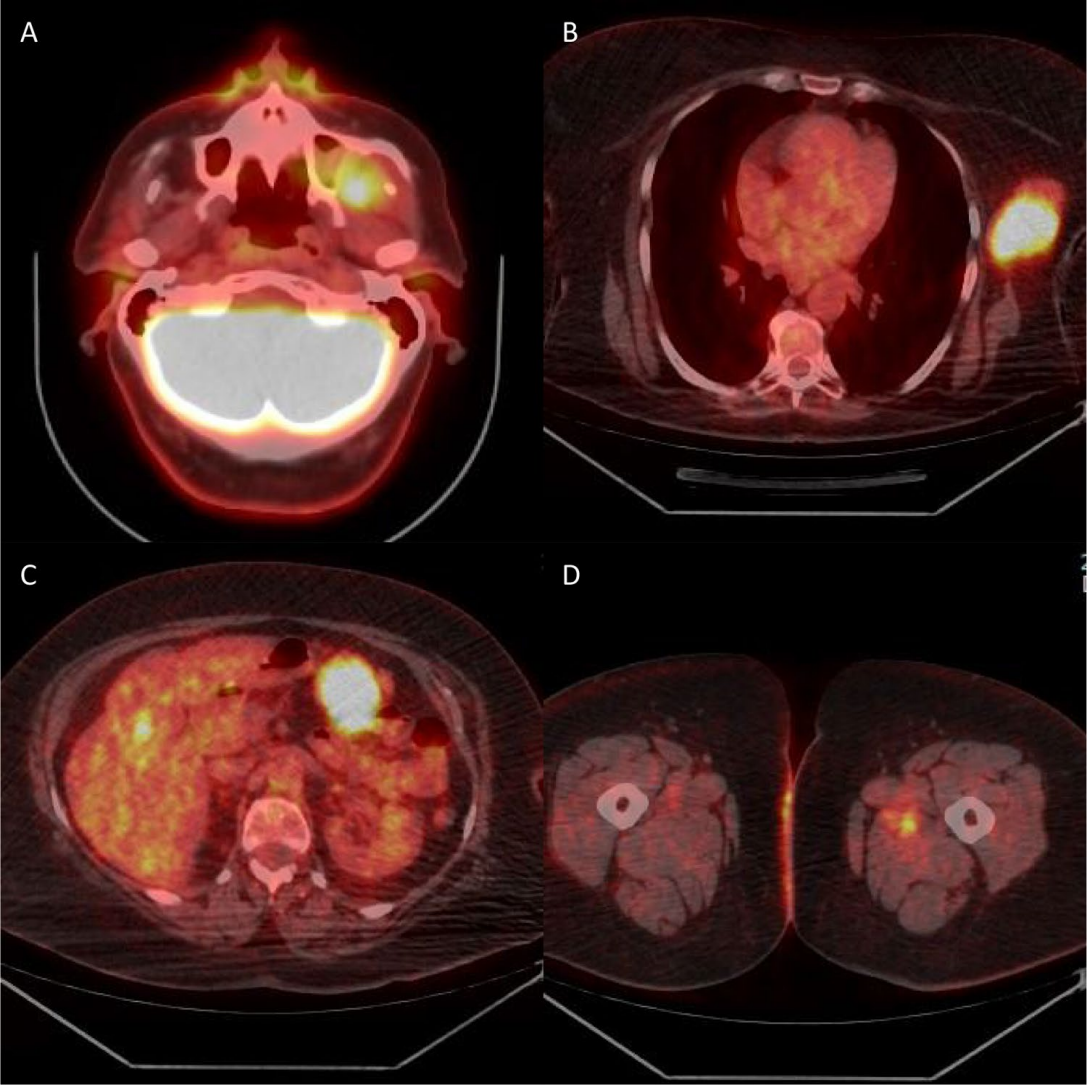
[F-18]FDG PET-CT reveals avid FDG uptake by the left buccal space lesion (a). Multiple pathological radiotracer hyper-accumulation localizations were present, such as in a voluminous left axillary lymph node (b), a perigastric solid lesion (c), and in the muscular tissue of the left thigh (d)
